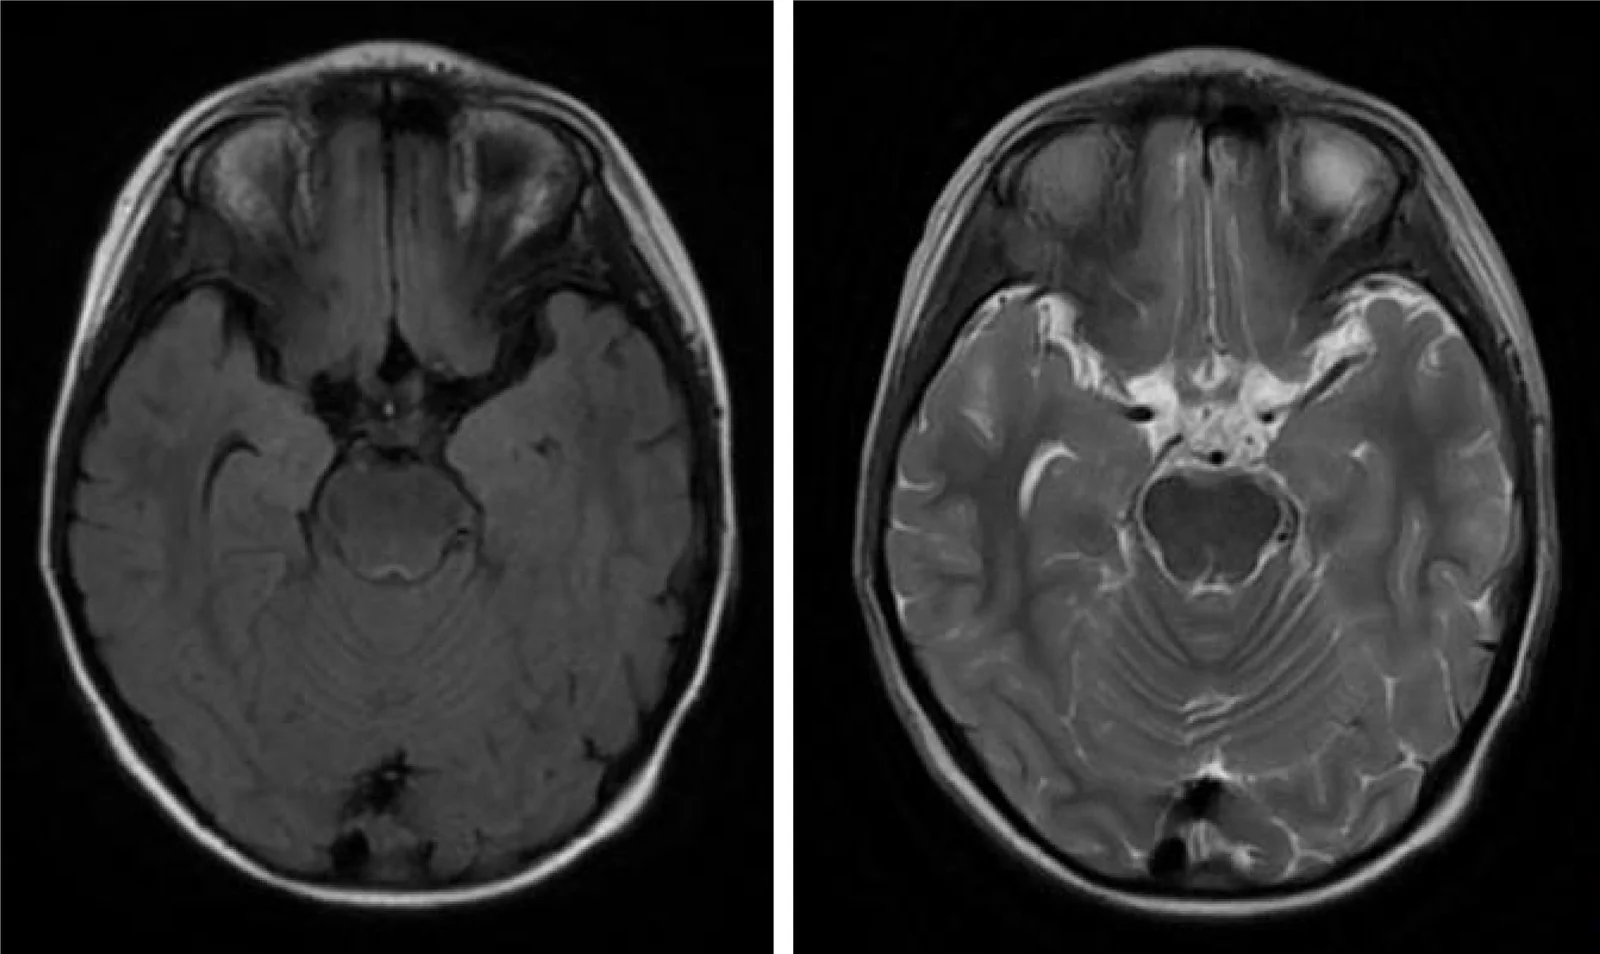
Brain magnetic resonance imaging for patient 4 in the acute phase. There is an increased intensity and edema in the left hippocampus and temporal lobe (T2 and T2 FLAIR).
